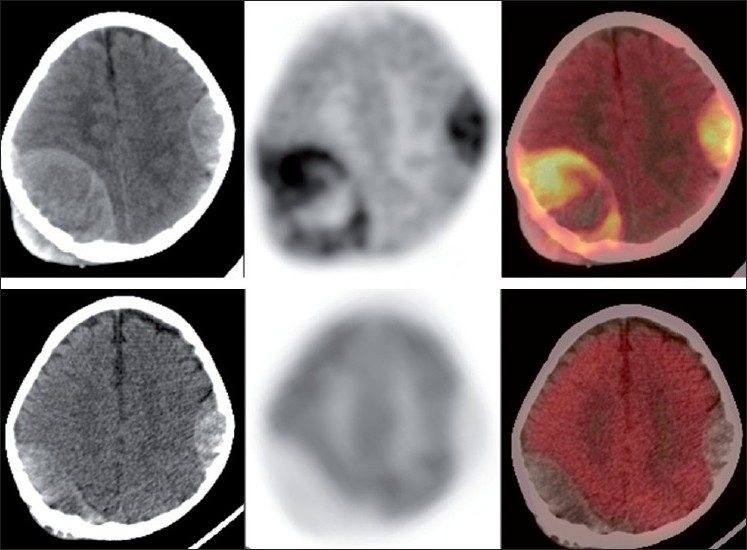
Transaxial CT, PET and PET-CT images (L-R) images of a patient with NB. Baseline scan (upper row) shows presence of meningeal metastasis. Scan done post two cycles of NACT (lower row) shows significant reduction in the FDG uptake. These finding are suggestive significant treatment response on PET-CT but partial significant response on CT
